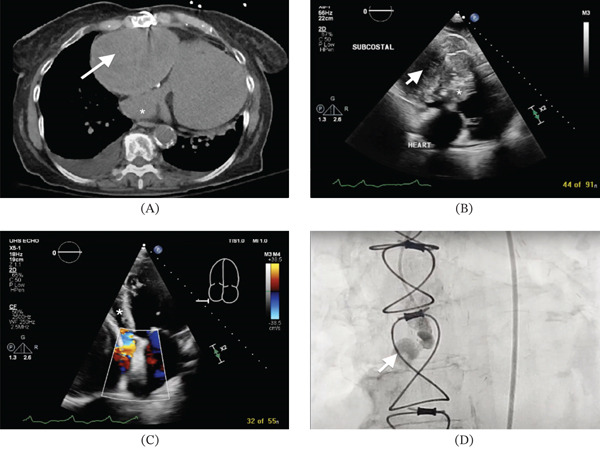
(A) Computed tomography image of the thorax showing a large 10 cm pseudoaneurysm (arrow) compressing the right ventricle (asterisk) and displacing the heart to the left. (B) Echocardiogram with a subcostal view showing a large pseudoaneurysm (arrow) causing compression of the right ventricle (asterisk). (C) Echocardiogram with a four‐chamber view showing right ventricular compression (asterisk) with minimal flow. (D) Coronary angiogram obtained from right coronary injection showing filling of the large pseudoaneurysm (arrow) of the SVG‐PDA conduit with no distal runoff.
